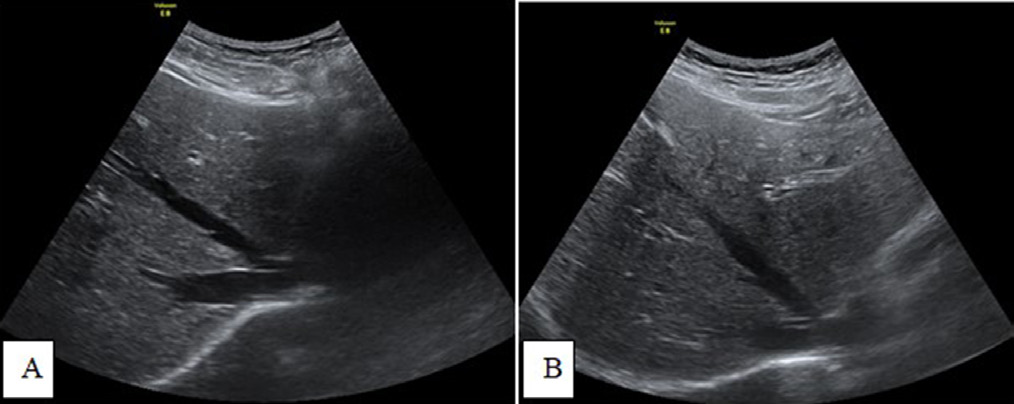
Transverse-axis ultrasonography images (A and B) showing an absent
